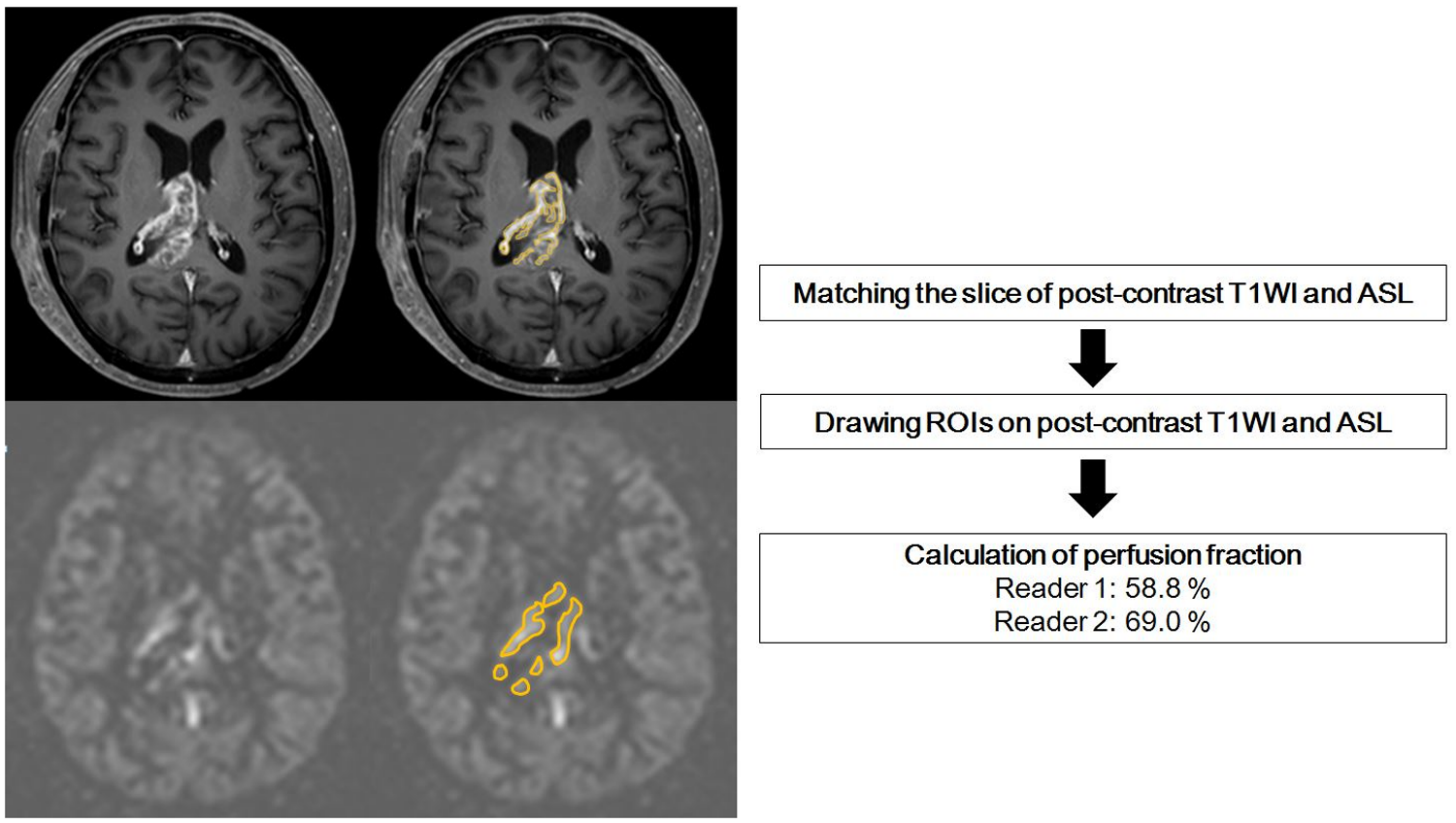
Accrual process for determining the perfusion status of surgical cavity wall enhancements. The perfusion fraction was calculated by dividing the area of the high perfusion on ASL MR imaging by the area of contrast-enhancement on postcontrast T1-weighted imaging. The perfusion fraction was 58.8% for reader 1 and 69% for reader 2.
